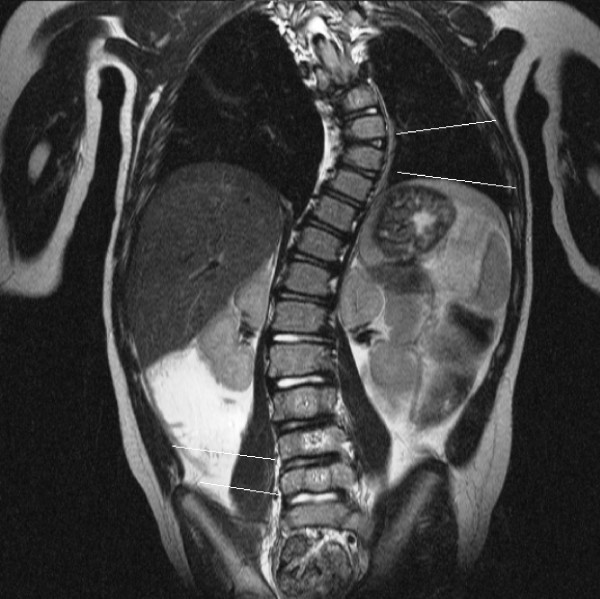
MRI T2-weighted Coronal Plane Image Idiopathic Scoliosis Patient #26. Schmorl's nodes on superior and inferior marker lines compared to normal discs at the adjacent levels.
